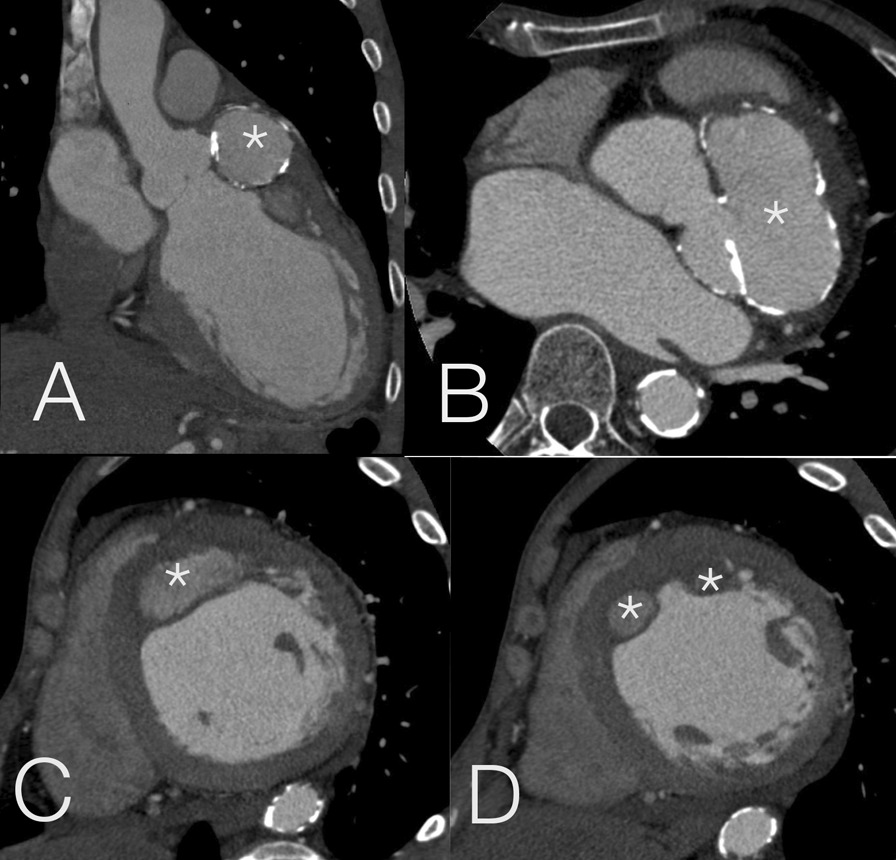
Cardiac computed tomography (CT). Left sinus of Valsalva aneurysm (LSVA) visualized using cardiac CT coronal (a) and axial (b) views, pointed by asterisks. The calcified wall of LSVA protruded and extended, forming myocardial dissection at the basal (c) to mid (d) anterior and anteroseptal walls, annotated with asterisks
